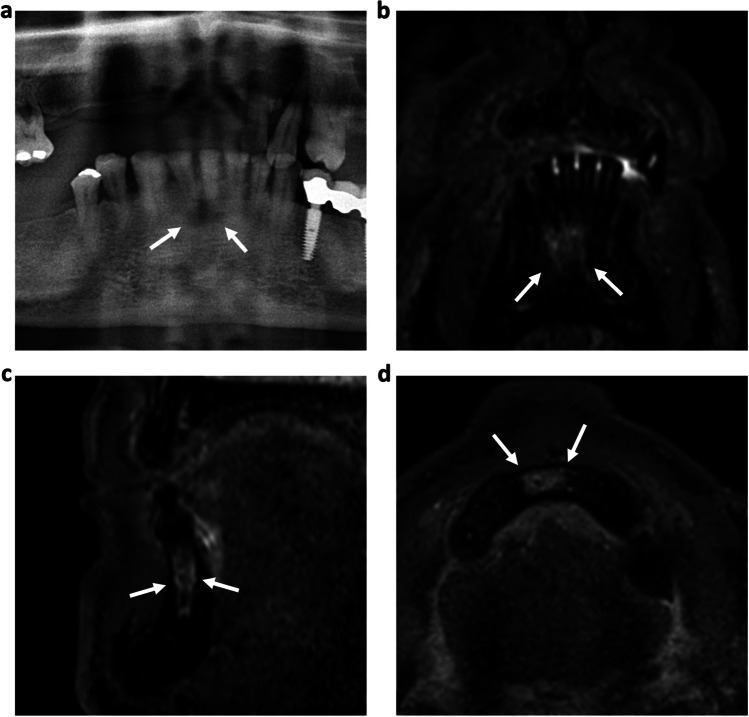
53-year-old patient with symptomatic periodontitis of the tooth 41. A distinctive periapical radiolucency (white arrows) can be seen on OPT (a) and dental radiograph (b). The coronal (c) and axial (d) reconstructions of a 3D STIR sequence show a larger bone marrow edema around the periapical lesion, indicating a larger inflammatory reaction (white arrows)
